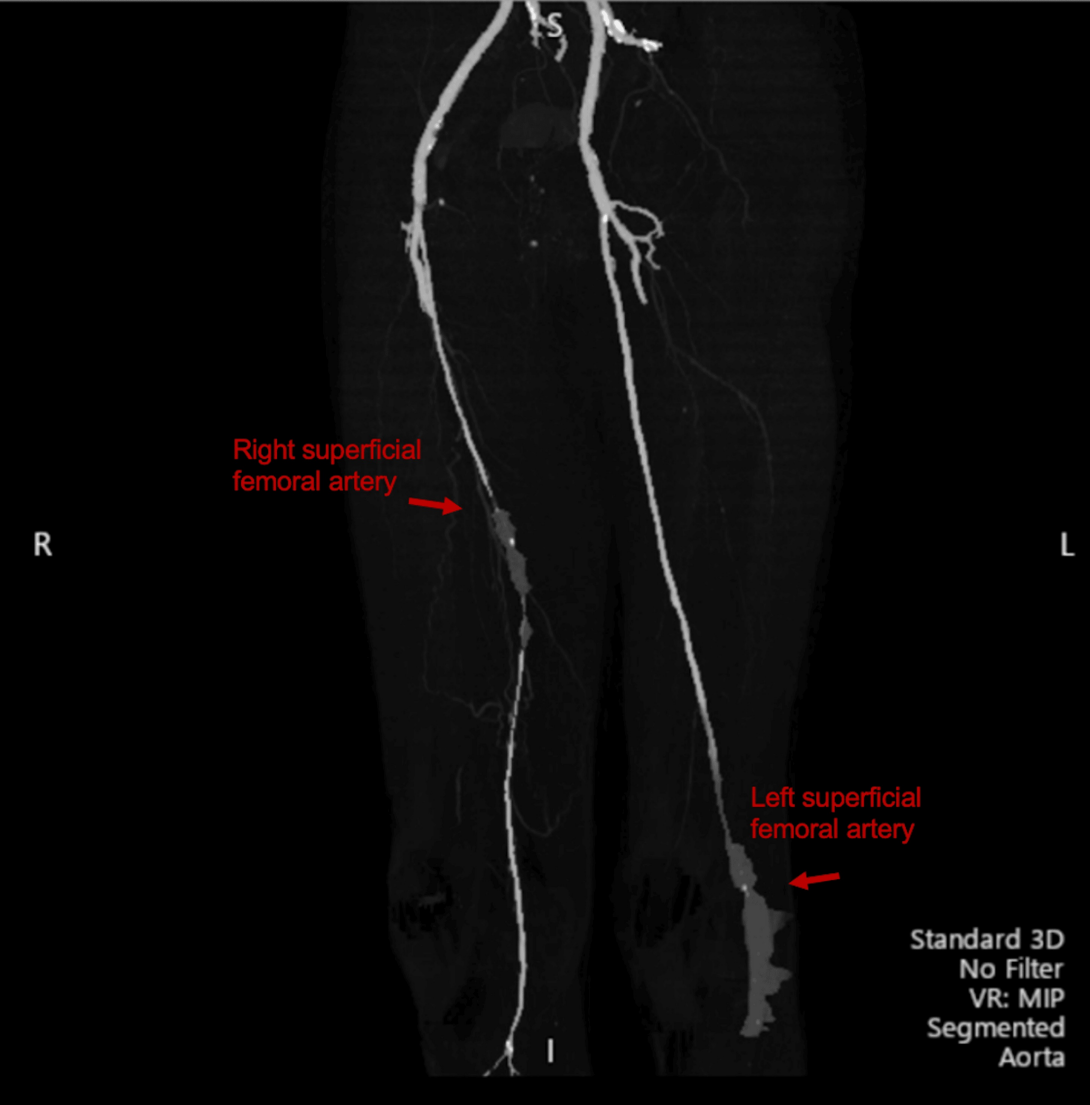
CT-angiography scan findings. Revealed greater occlusion of the right femoral profunda femoris artery in the right leg, compared to the thrombus occlusion of the left cold limb. Occlusion of the left profunda and popliteal artery without distal perfusion in the left leg is suspicious for a mixed calcified thromboembolic source.
